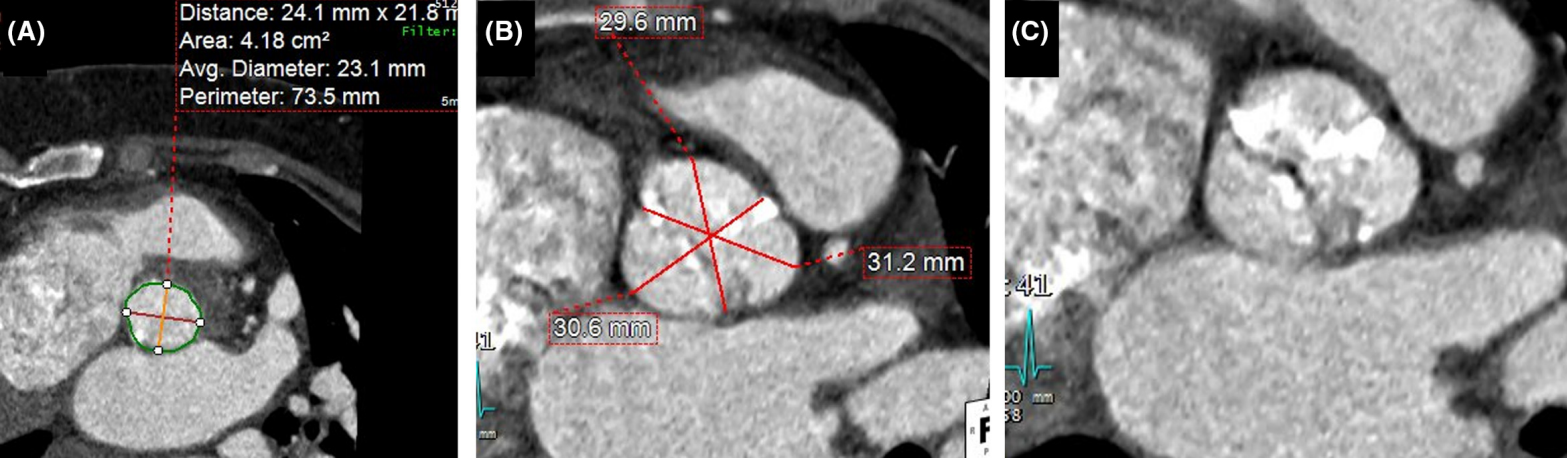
Cardiac CT images of valve preprocedure. (A) Measurements at annulus level. (B) Measurements at sinus level. (C) The bicuspid morphology of valve leaflets.
